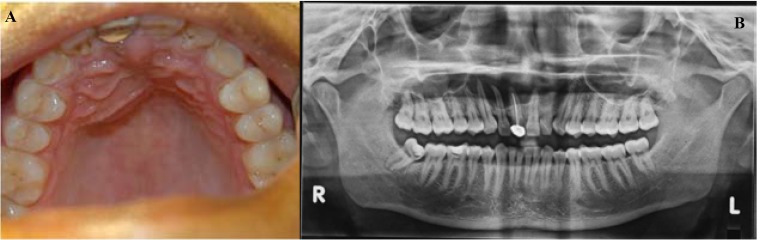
A.-Palatal swelling. B.-Orthopantomogram.
